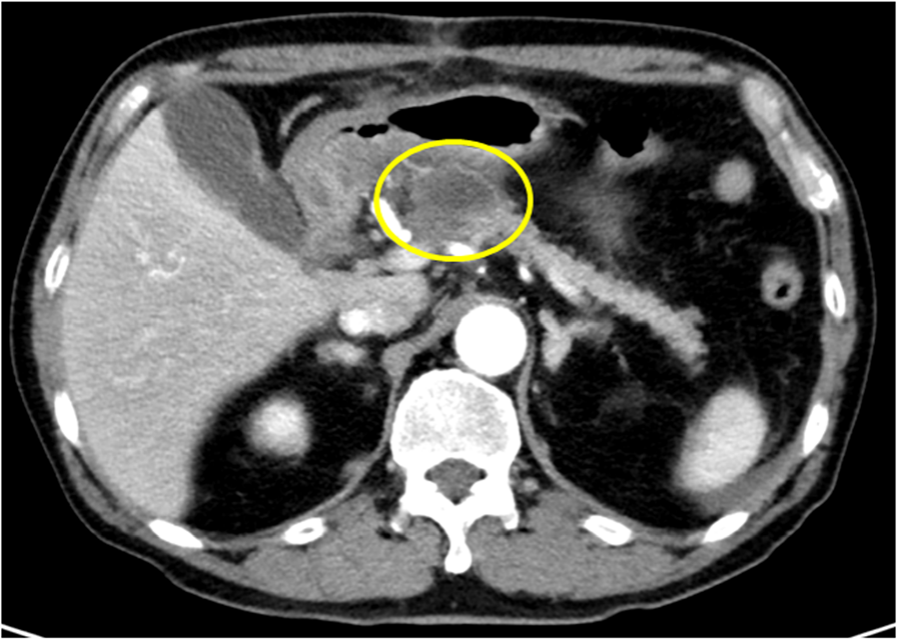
Pancreatic pseudocyst. Acute pancreatitis repeatedly occurred 1 month after the first operation, and a pancreatic pseudocyst developed at the same site. Enhanced computed tomography (CT) showed a cyst with a diameter of 29 mm in the body of the pancreas
